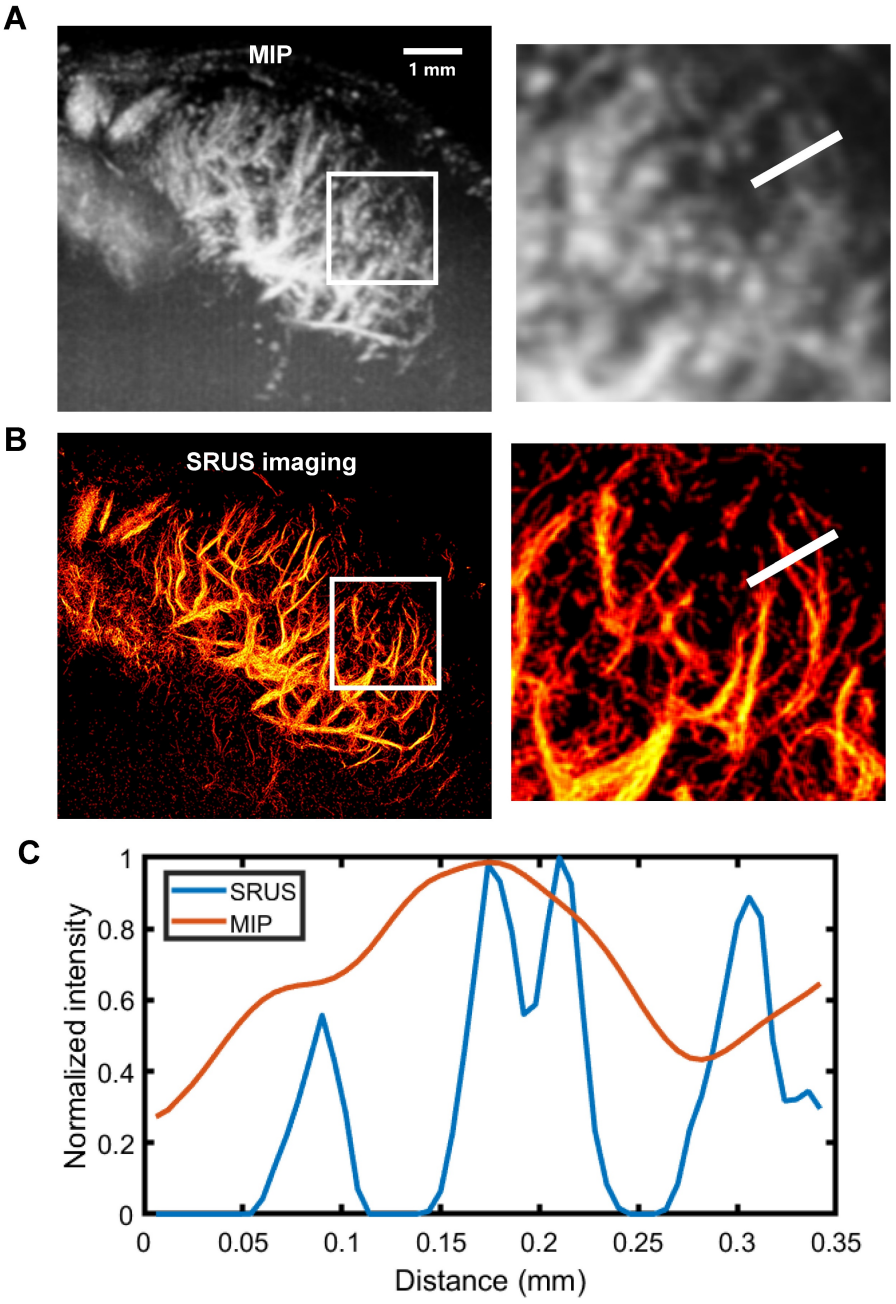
SRUS imaging and spatial resolution quantification of a single lymph node cross-section. (A) Maximum intensity projection (MIP) derived from a 1,500- contrast-enhanced ultrasound (CEUS) images. Local regions of interest (white rectangles) are enlarged for detailed comparison. (B) Reconstructed microvascular map using super-resolution ultrasound (SRUS) imaging. (C) Pixel intensity profile across the sampled region (white lines in A and B).
